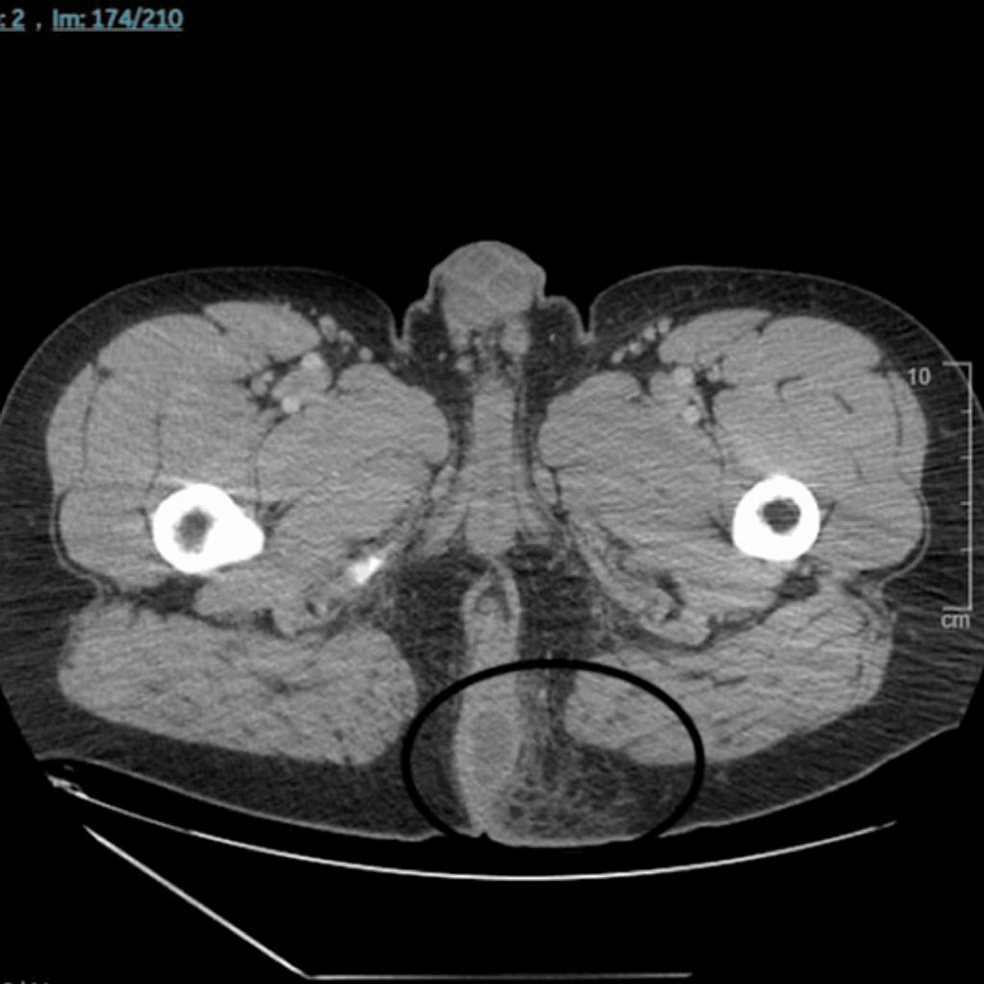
CT abdomen and pelvis w/ IV contrast showing left perianal abscess in the subperitoneal space measuring 3.4 cm x 1.9 cm (black circle), with stranding in the adjacent soft tissues
